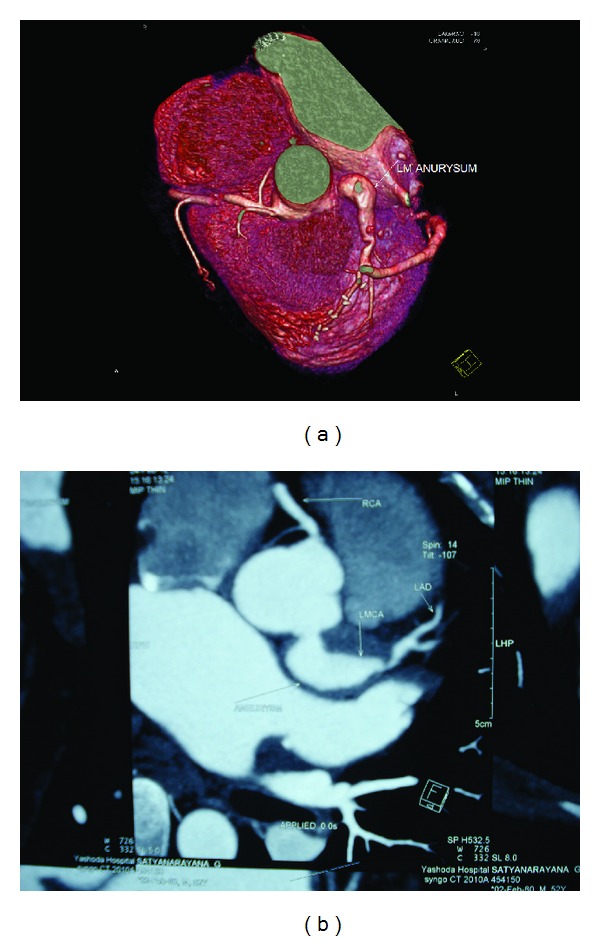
(a) CT Coronary angiogram showing left main aneurysm with ostial LAD stenosis. (b) CT Coronary angiogram showing left main aneurysm with ostial LAD stenosis.
